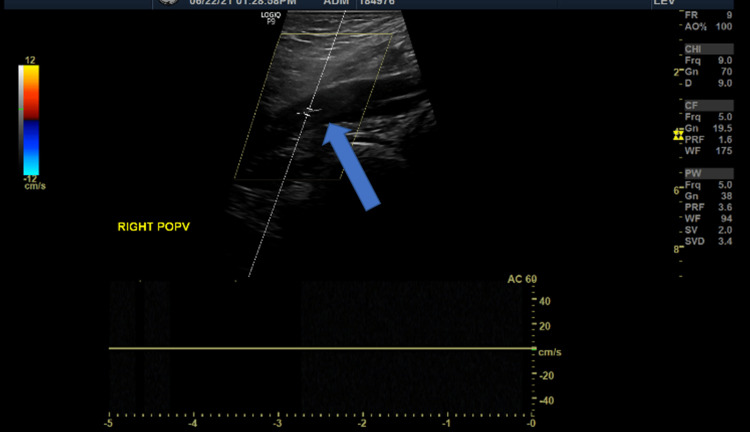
Doppler image shows non-compressible right popliteal vein with absent blood flow (blue arrow)
